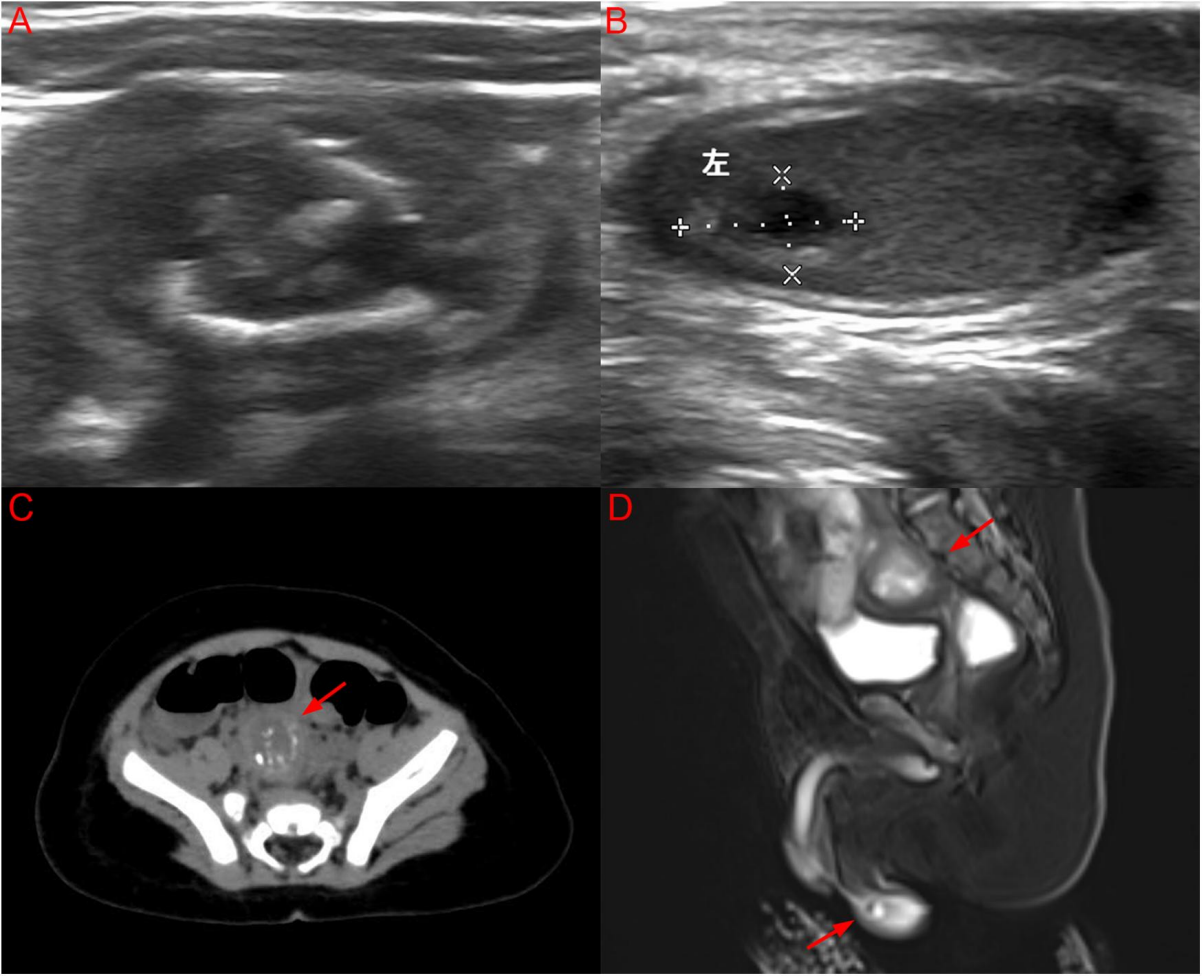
(A) US image of a well-defined mixed echogenic mass in the lower right abdomen; (B) US image of a cystic solid mass lesion in the left testis; (C) CT image of a mixed density mass located above the bladder with an arrow; (D) MRI image of a right IAT with a mixed mass and a mass in the left testis synchronously with arrows. (Color version available online.).
